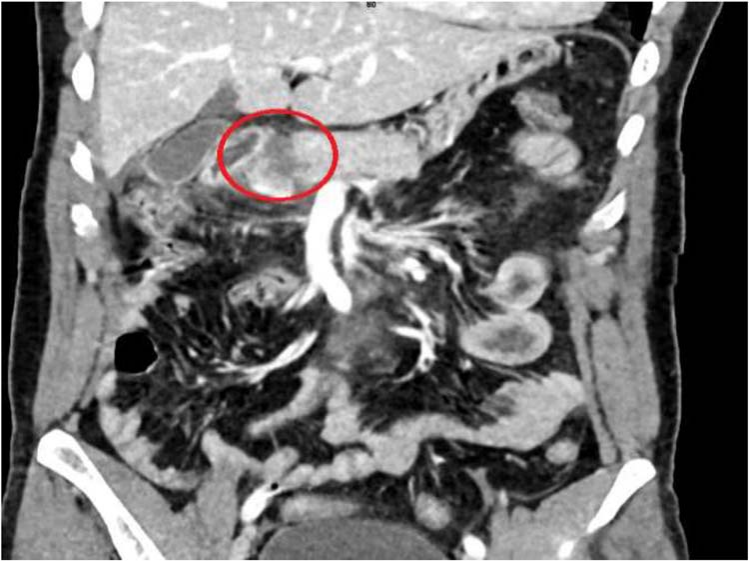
CT abdomen—coronal section, showing transection of the pancreas (circle).
